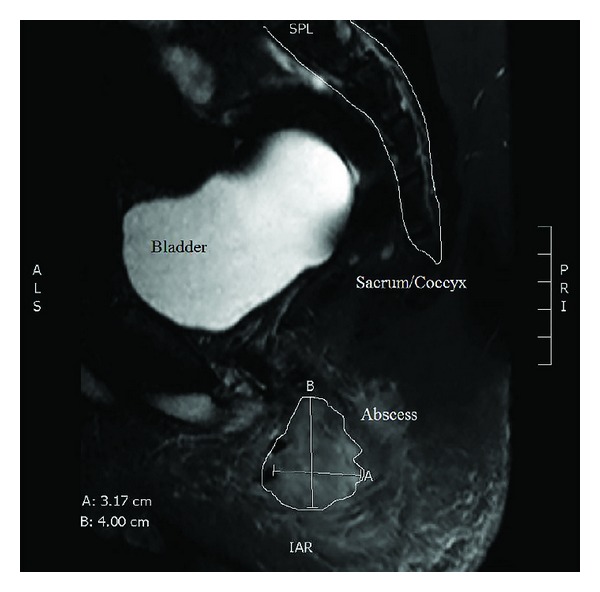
T2 MRI, sagittal view sacrum/coccyx. Magnetic resonance imaging revealing an approximately 3.0 × 4.0 cm phlegmon consistent with perirectal abscess.
